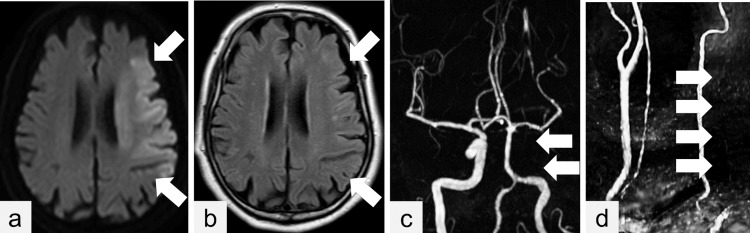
Head magnetic resonance imaging (a) Head magnetic resonance imaging shows increased signal intensity in the left middle cerebral artery territory on diffusion-weighted imaging (arrows). (b) This region does not exhibit increased signal intensity on fluid-attenuated inversion recovery imaging (arrows). (c and d) Magnetic resonance angiography shows an absence of flow in the left common carotid artery and internal carotid artery (arrows).
